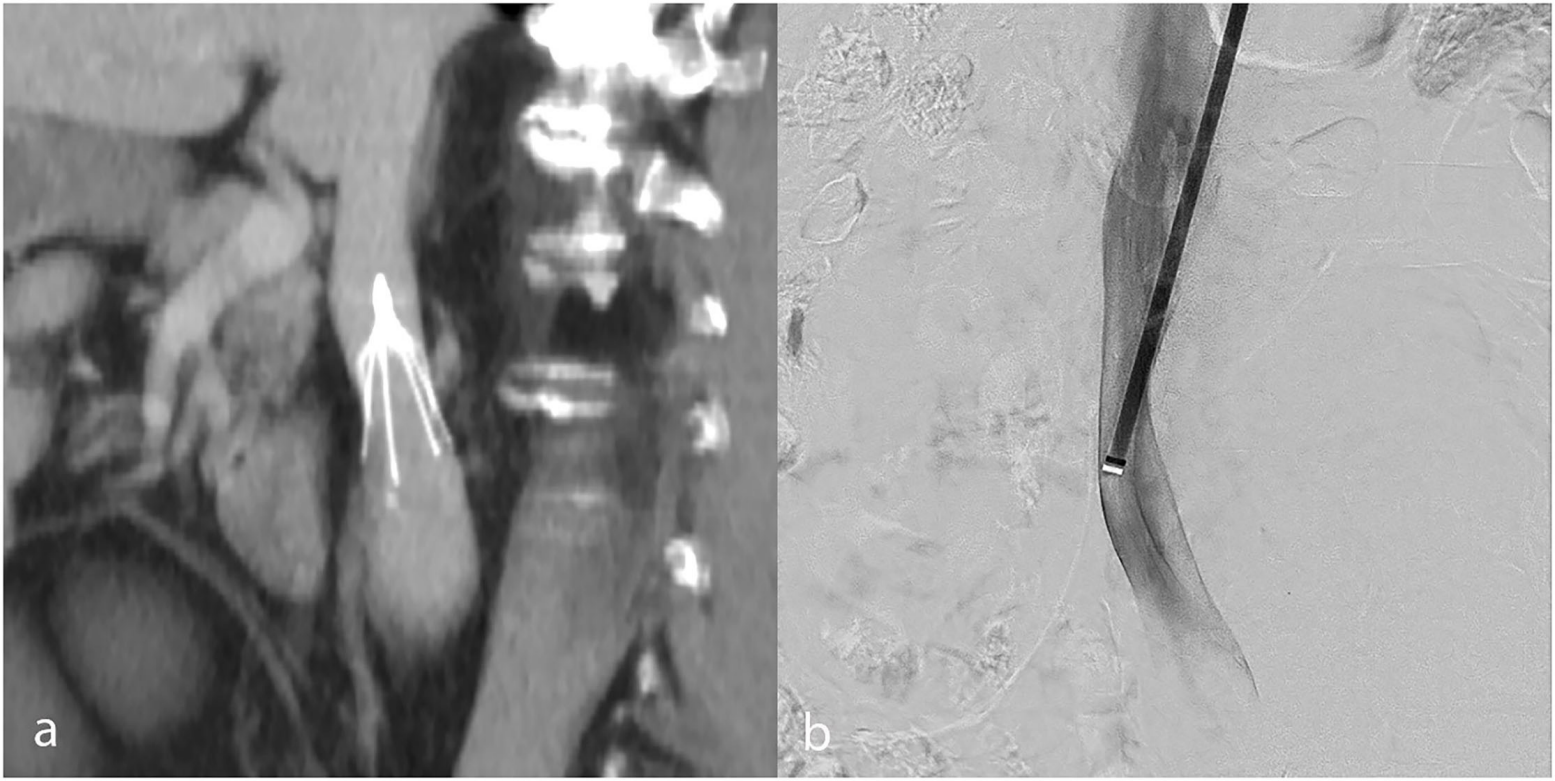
IVC stenosis due to IVCF use, shown in a patient on coronally reformatted pre-retrieval CT venography (a). Post-retrieval subtracted catheter-directed cavography (b) confirms a persistent focal IVC stenosis at the former filter site.
